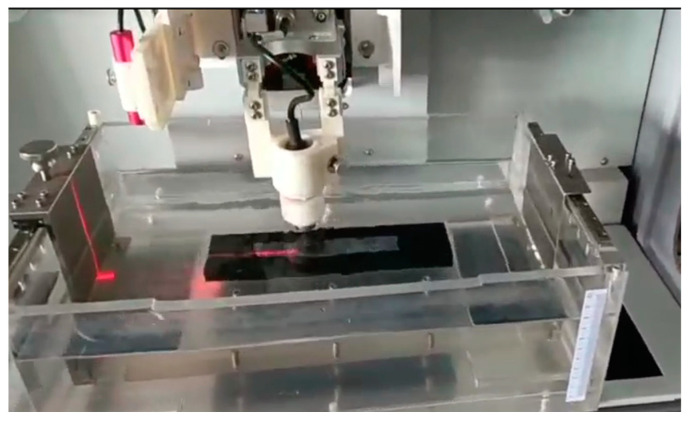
Water immersion ultrasonic testing system.
